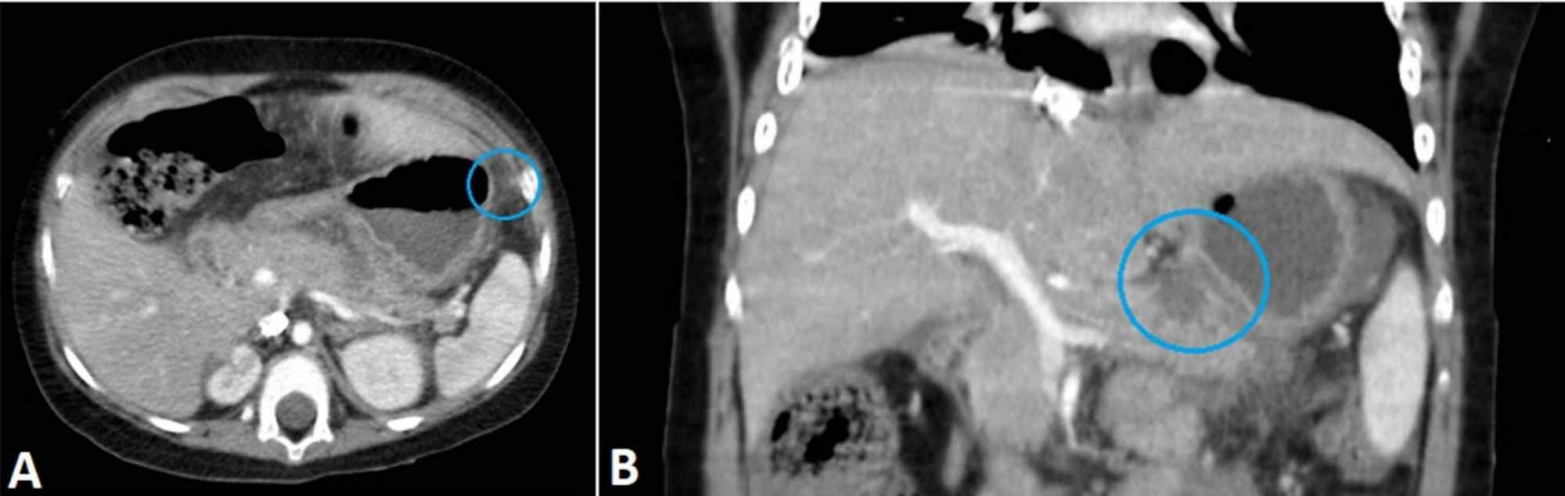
A CT examination suggestive of necrotizing pancreatitis. (A) Axial view. (B) Coronal view. CT, computed tomography
